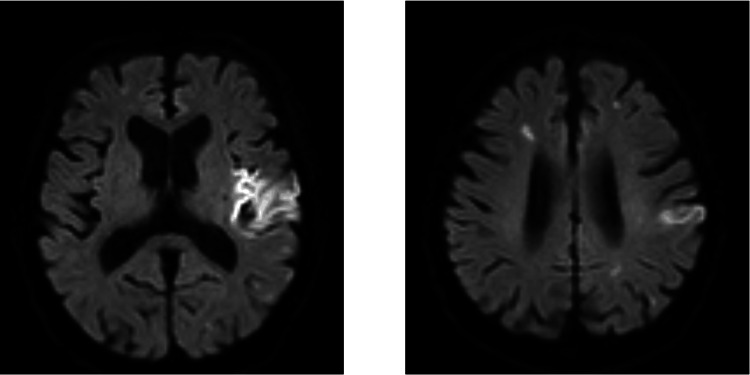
Findings of magnetic resonance imaging (MRI) in case 2 MRI scan reveals multiple cerebral infarctions (diffusion-weighted MRI scan), which developed six days after the first nivolumab administration.
